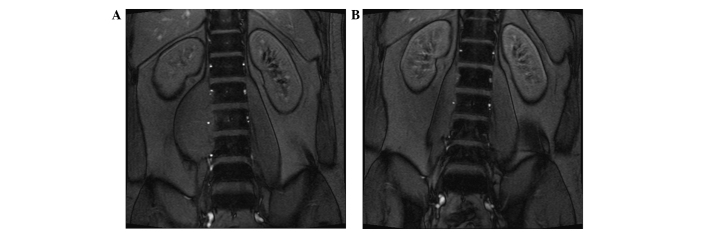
Magnetic resonance imaging of the lumbar myeloid sarcoma (A) prior to and (B) subsequent to the combined therapy.
